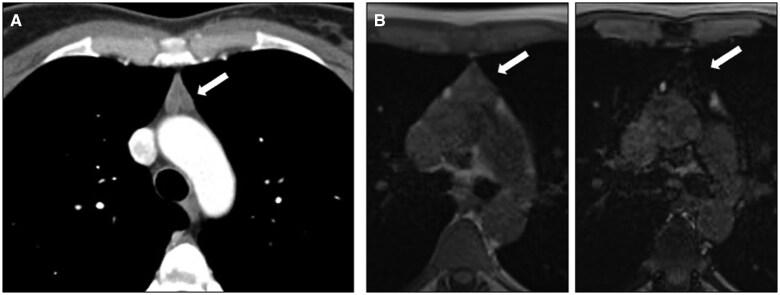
Thymic hyperplasia. (A) Contrast-enhanced axial CT shows a diffuse enlargement of the thymus (arrow). (B) Chemical-shift MR imaging demonstrates in-phase homogeneous intermediate signal intensity of the thymus (left) and signal drop on out-of-phase imaging (right), consistent with thymic hyperplasia.
